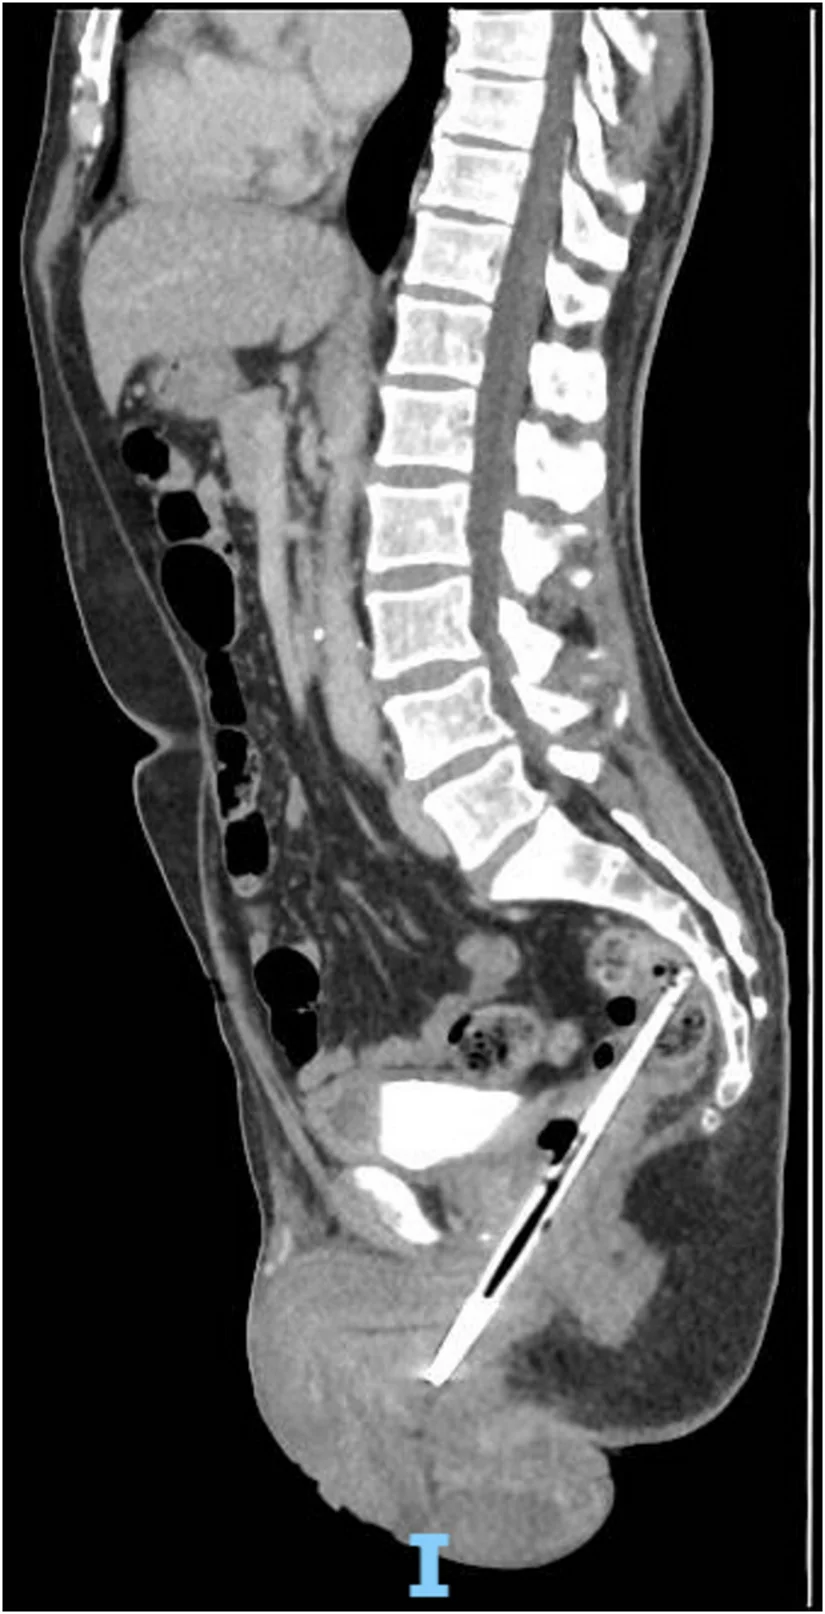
Sagittal CT slice demonstrating the paintbrush penetrating the bulbar urethral through the rectum and terminating at the rectosigmoid junction without perforation into the presacral space. CT = computed tomography
